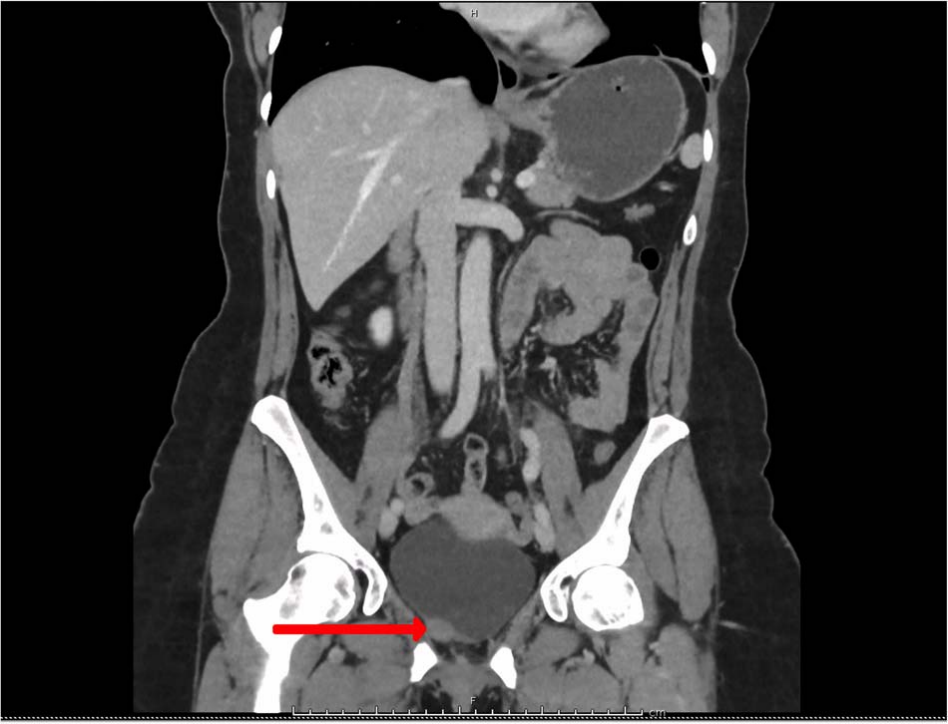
CT urogram (coronal view) revealing a 1.5 cm thickening at the level of the right lateral bladder neck and a 1-cm-soft tissue thickening at the bladder dome.
